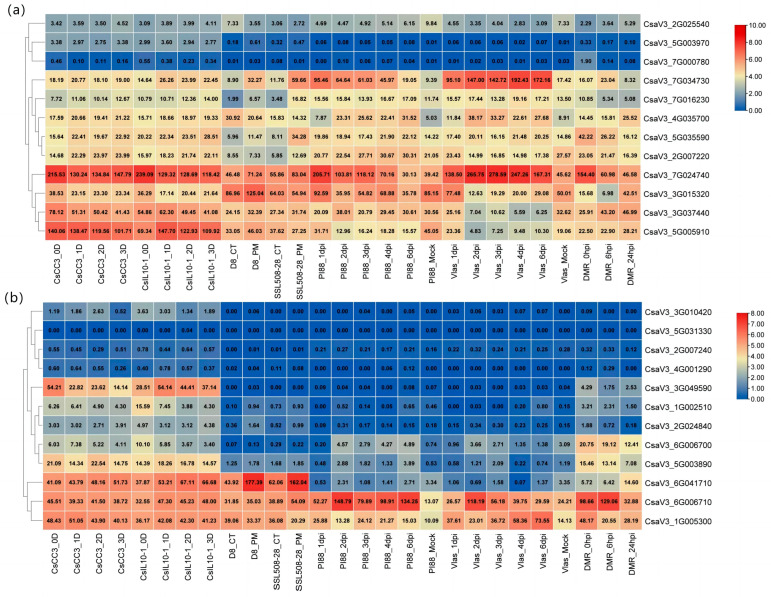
Expression profile of cucumber genes *CSINVs* (a) and *CSINHs* (b) under biotic stress. CsCC3 refers to nematode-resistant varieties, CsIL10 refers to non-insect-resistant varieties, and the following numbers indicate the days after infection with nematodes. CT refers to mock as a control, PM refers to powdery mildew, D8 is the recurrent parent, and SSL508-28 is the powdery mildew-resistant segment substitution line. PI88 refers to downy mildew-resistant varieties, Vlas is susceptible to downy mildew varieties, and the numbers indicate the days of downy mildew infection. DMR refers to downy mildew resistance, and hpi refers to hours post-infection. The data in the boxes represent raw RPKM values.
